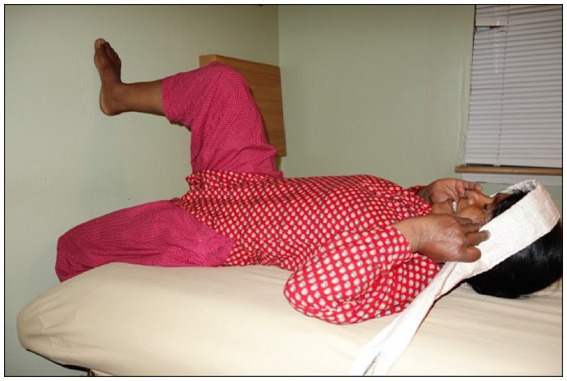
Positioning of participants for ultrasound and pressure data collection for the six tasks.
